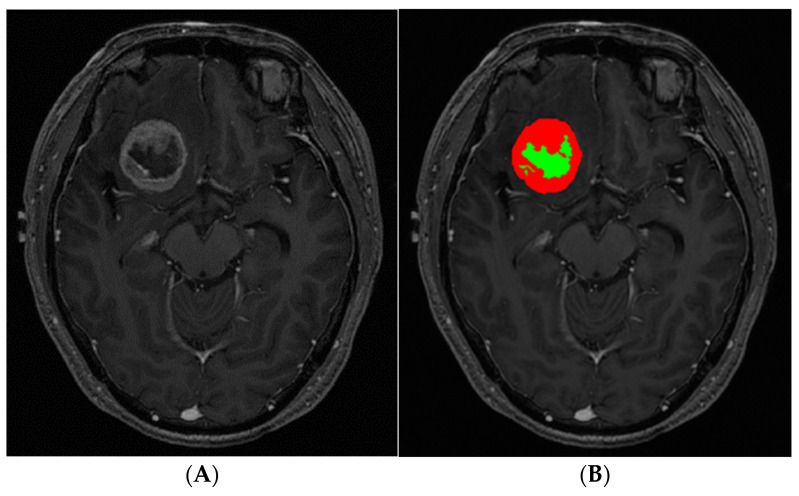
Use of a semiautomatic method to segment brain metastasis (BM) as regions of interest in a 39-year-old man with brain metastasis from lung cancer. (A) Contrast-enhanced T1-weighted magnetic resonance (MR) image. (B) Enhancing lesions (solid, red) and non-enhancing lesions (necrosis, yellow) are segmented separately.
